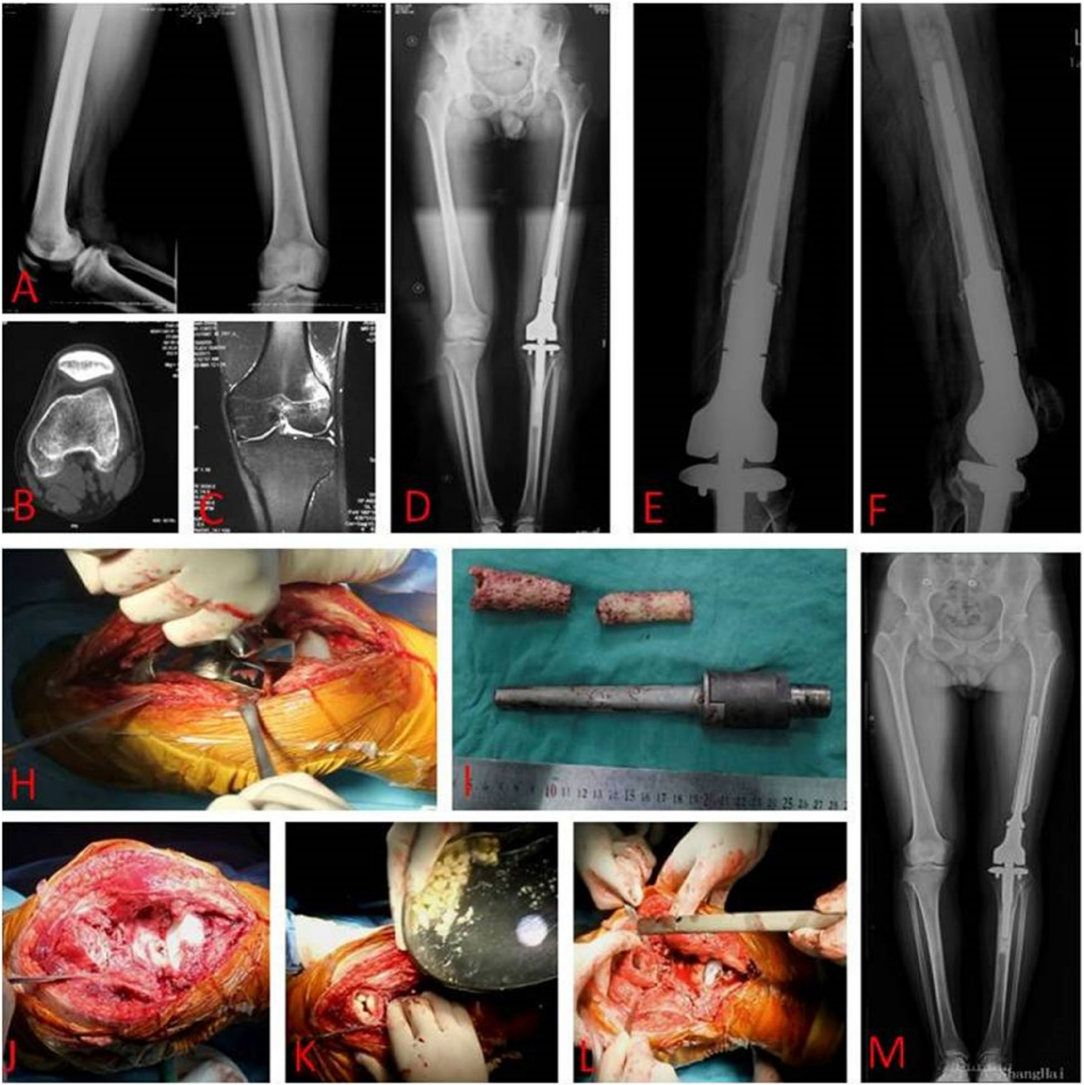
A 22-year-old osteosarcoma male patient, underwent a revision surgery because of the prostheses loosening. A–C Preoperative X-ray, CT-scan, and MRI image showed a lesion at the distal femur. D Postoperative 1-year X-ray showed the prosthetic components at position. E, F Postoperative 3-year X-ray showed a prostheses loosening at the femur site. H, I, Prosthetic components at the femur site were removed. J, K The residual cement was removed and followed with allograft implanted. L A biological prosthetic component was implanted. M Postoperative full length film of double lower extremities showed the prostheses at position
